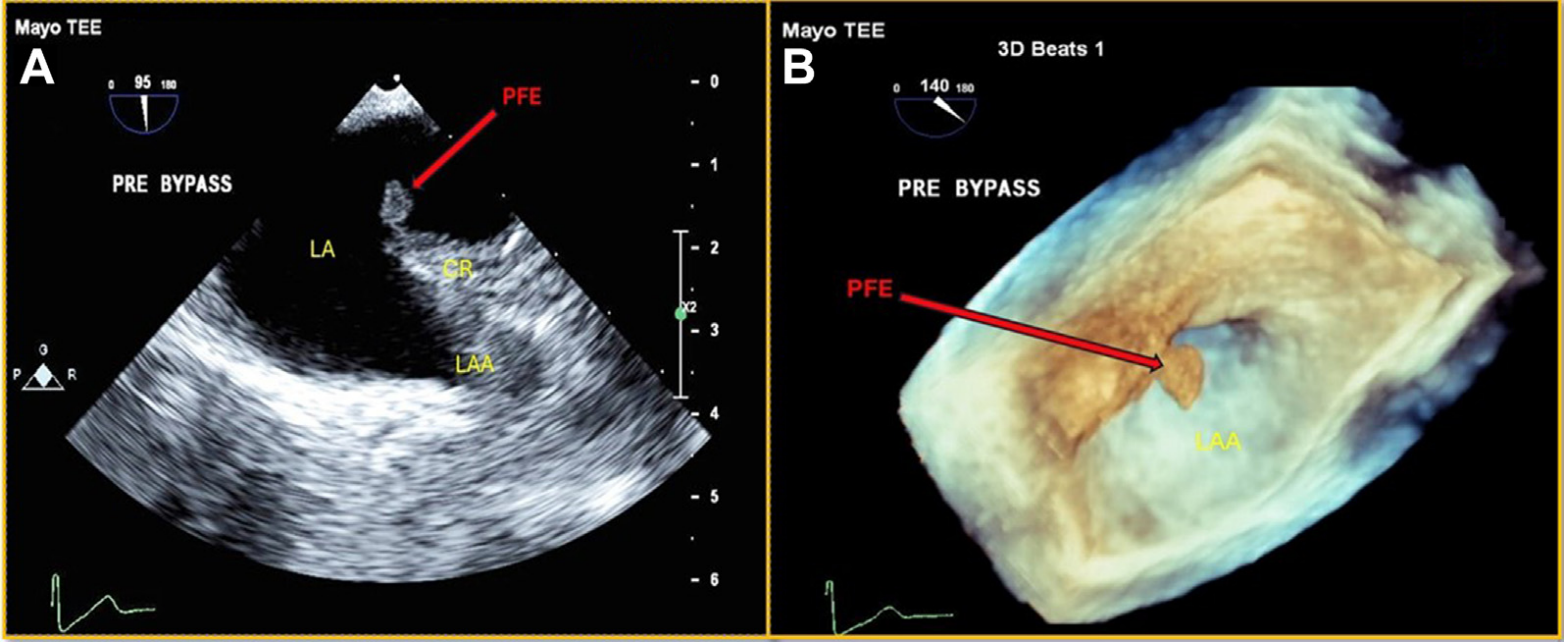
Transesophageal Echo (A) TEE, 2-D mode-focus on LAA, showing a PFE on the coumadin ridge at the apex of the reflection of LAA and LUPV tissue. (B) 3-D transesophageal echo of a PFE attached to the orifice of the LAA en face. LUPV = left upper pulmonary vein; other abbreviations as in Figures 1 and 2.
